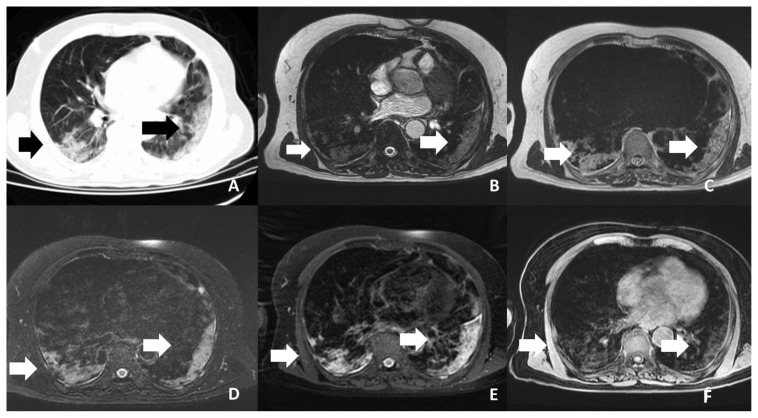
Shows bilaterally peripherally located ground glass and consolidation areas of the patient. A-CT B-FIESTA sequence C-SSFSE sequence D-T2 FS sequence E-T2 PROPELLER sequence F-T1 LAVA-F sequence
